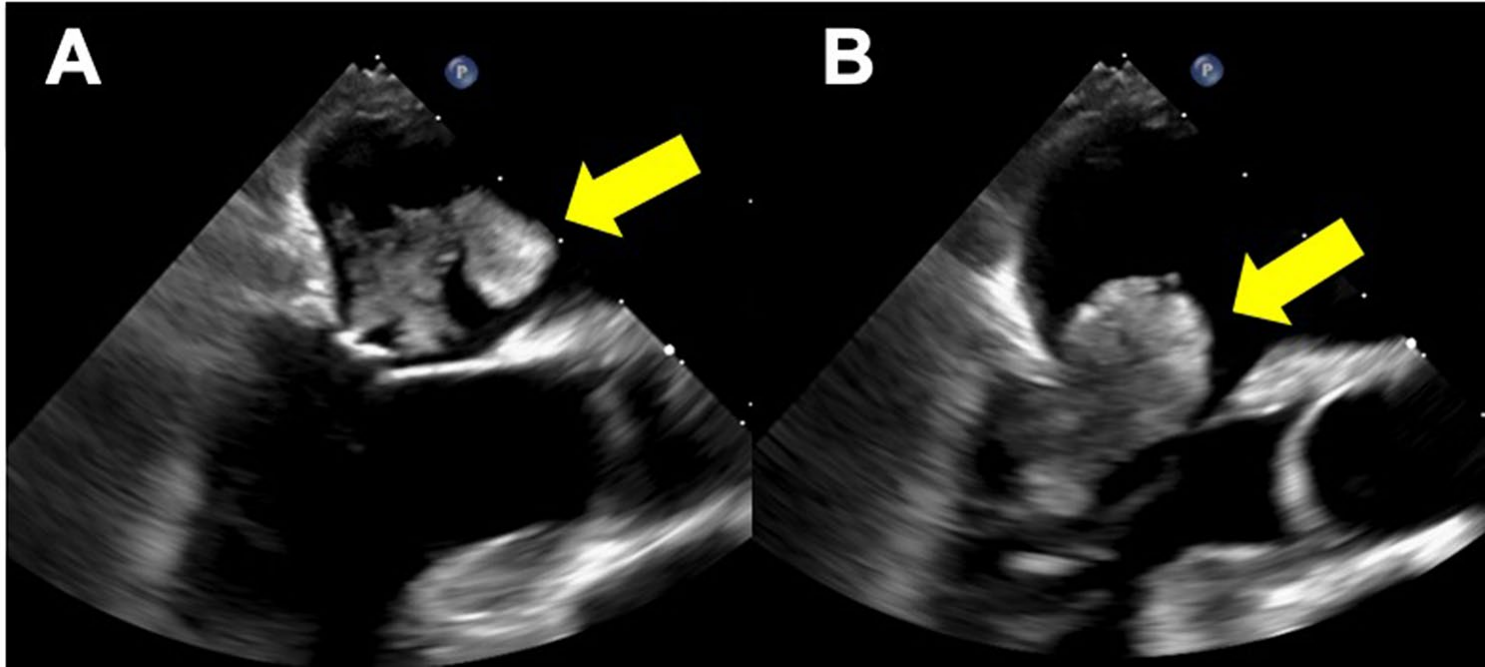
Preoperative transesophageal echocardiography. The left atrial tumor (yellow arrow) was attached to the anterior mitral leaflet. No significant mitral regurgitation was observed (A, B)
